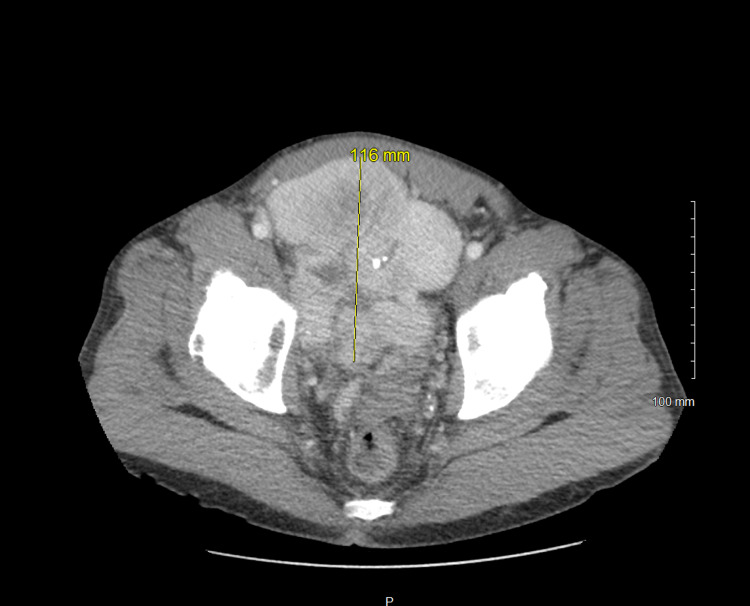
Computed tomography (CT) imaging of the abdomen and pelvis revealed a lobulated mass with heterogeneous echotexture in the anterior pelvis measuring 11.4 x 10.4 x 10.1 cm.
